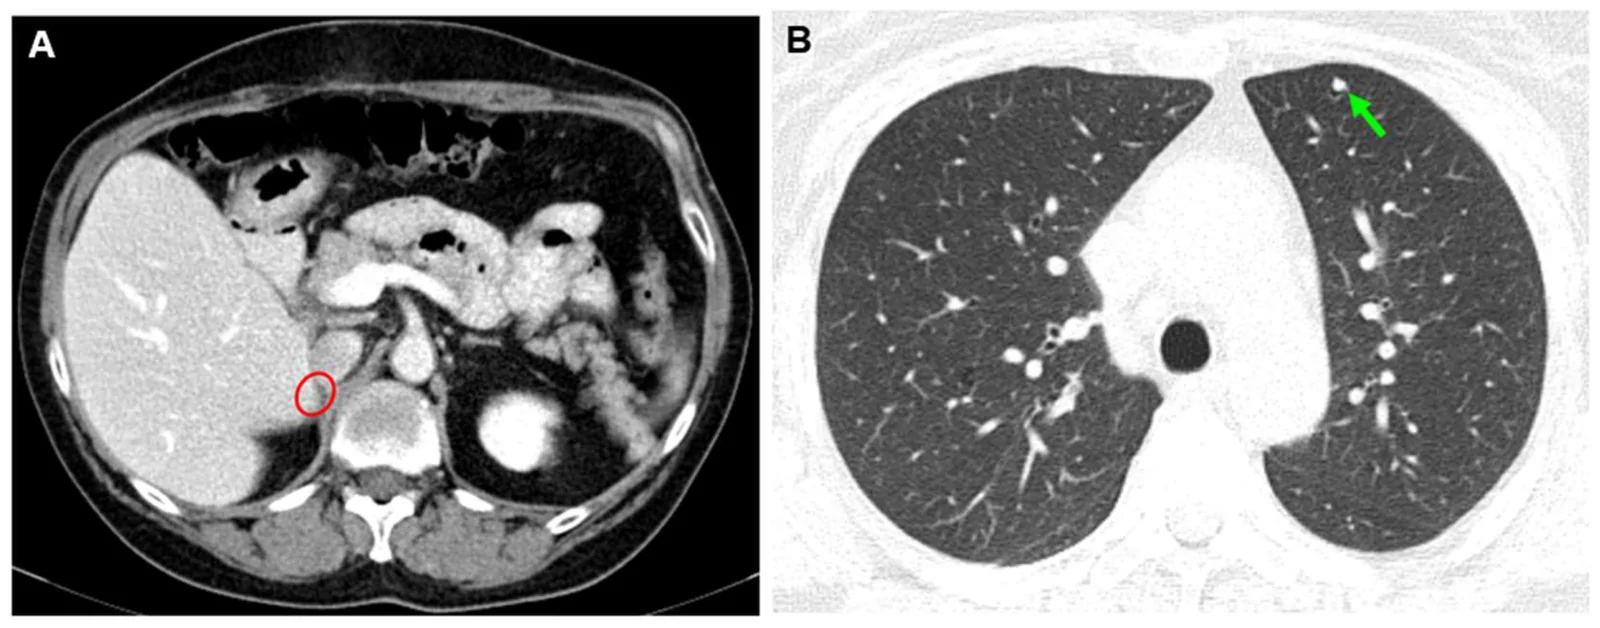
Within the year following thoracic surgery, the computed tomography (CT) showed no local recurrence at the pulmonary and abdominal intervention sites [(A) postsurgical fibrosis (red circle) at the place of right adrenalectomy (contrast-enhanced CT scan, axial plane, venous phase)]. In addition, a novel pulmonary micronodule of 4.7 mm in the anterior segment of the left upper lobe was detected on CT scan [(B) left upper lobe with micronodule (green arrow) at contrast-enhanced CT scan, axial plane, lung window].
